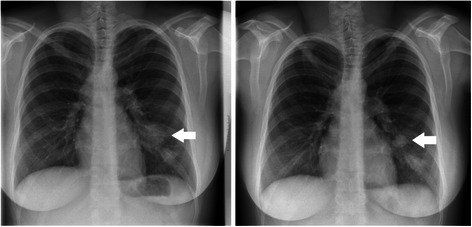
Chest X-ray examination before (left) and after (right) surgical abortion. The diameter of the largest pulmonary manifestation decreased significantly
